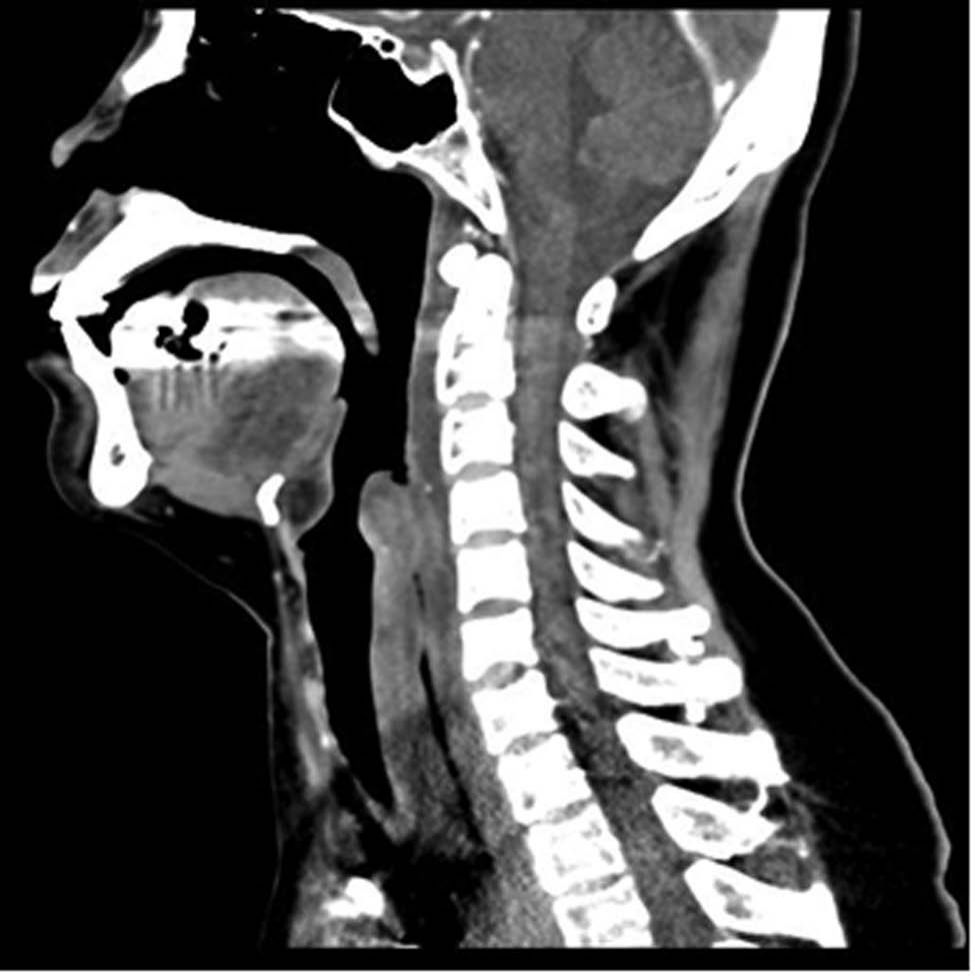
Contrast-enhanced chest computed tomography of a sagittal view reveal diffuse esophageal wall thickening with low attenuation and paraesophageal fluid collection in the mediastinum along the entire length of the esophagus.
